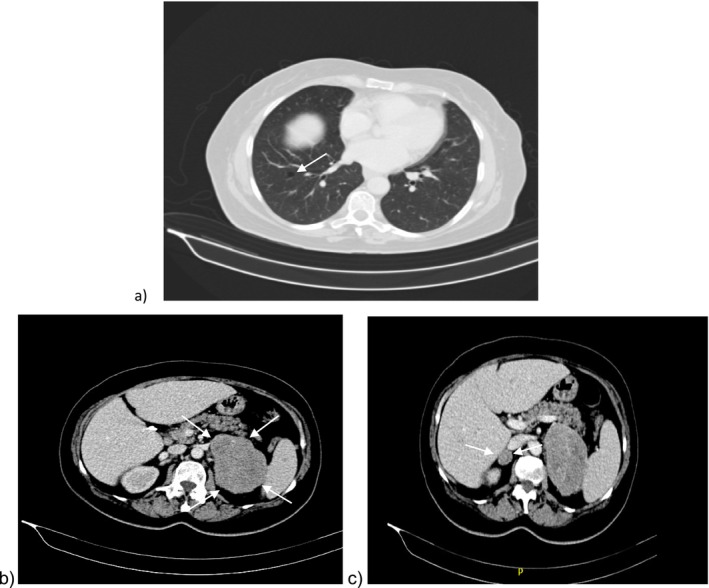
Initial computed tomography (CT) of the abdomen/pelvis. (a) 7 mm bleb in the right lower lobe most probably the origin of the tumor. (b) Large, heterogenous retroperitoneal mass arising from the left adrenal gland, measuring 14 × 9 cm in axial representation. (c) Large, heterogenous retroperitoneal mass arising from the left adrenal gland, measuring 14 × 9 cm and a controlateral 2 cm tumor arising from the right adrenal in axial representation. No magnification of any of the images was done. They were copied as they appeared on the viewer.
